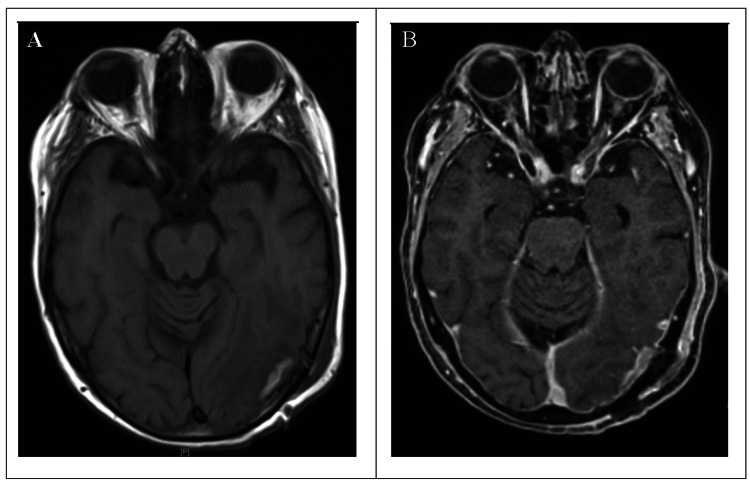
Imaging after surgical resection (A) Axial postoperative T1-weighted native MRI image. A small superficial hyperintense collection of blood is visible, but no relevant postoperative bleeding can be seen. (B) Axial postoperative T1-weighted MRI image with contrast, showing no relevant enhancement and gross total tumor resection.
